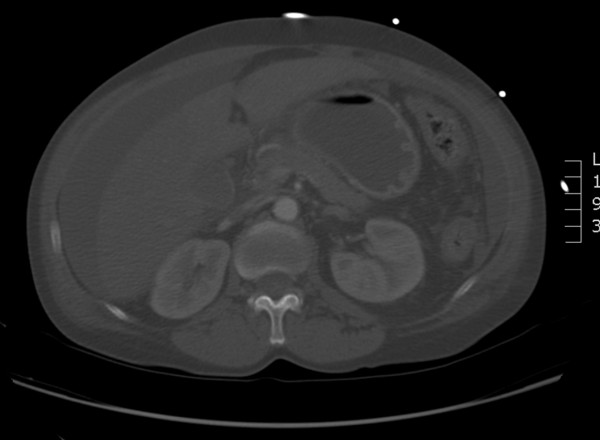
CT scan of the abdomen showing free peritoneal fluid.
